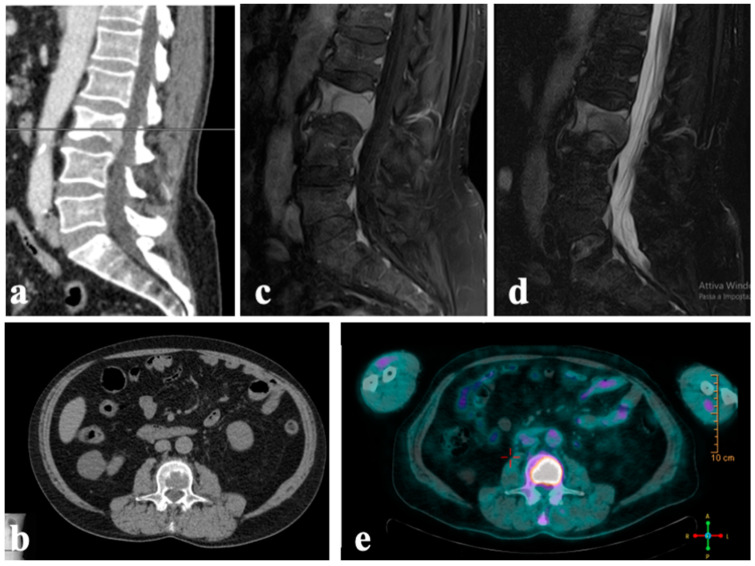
Case 1 at 3 months of follow-up: (a) Sagittal and (b) corresponding axial CT scans show a lytic lesion in the L3 vertebra with posterior erosion of the cortex, involving the spinal canal. (c) Sagittal T1-weighted MR imaging with contrast medium shows a homogeneous lesion at the L3 level with mixed hyperintense signal, whereas in (d) sagittal T2-weighted MRI, the lesion appears hypointense. (e) FDG-PET/CT on axial view shows the intense focal area of 18-FDG activity in the L3 vertebral body.
